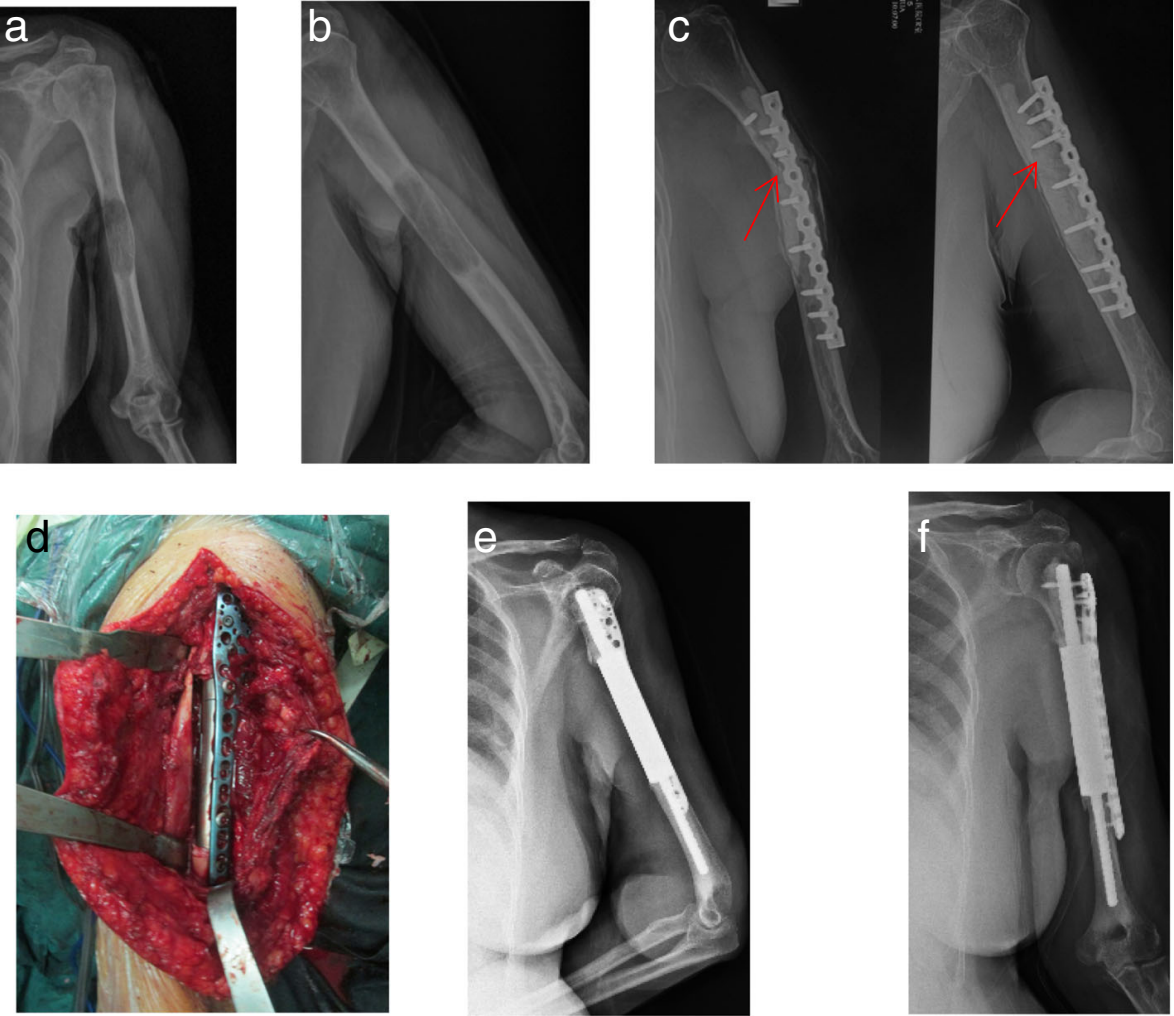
Case 20, a 62-y old female patient with osteolytic lesion due to multiple myeloma in the left humerus. The preoperative bone strength score is 12. a, b The tumor was located in the left humerus shaft (S5). c The patient underwent resection of the tumor and steel plate osteosynthesis with adjunctive bone cement. Fracture and screw breakage occurred 33 months after surgery (arrow). d Then, this patient underwent removement of the internal fixation and intercalary prosthetic reconstruction with an additional plate. e, f Postoperative X-ray indicated that intercalary prosthesis was in excellent position
